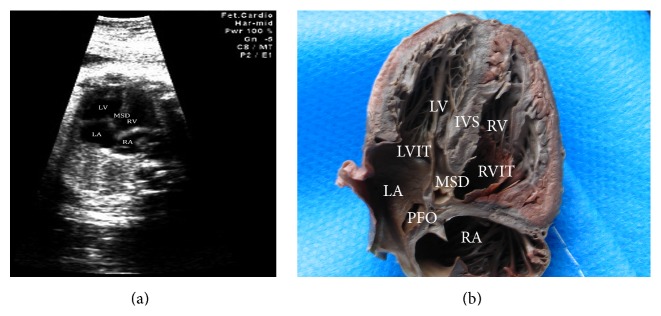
Ventricular septal defect (VSD) (32 w). (a) The apex four-chamber echocardiography section. (b) The apex four-chamber sectional anatomy. LA: left atrium; LV: left ventricle; RA: right atrium; RV: right ventricle; PFO: patent foramen ovale; IVS: interventricular septum; LVIT: left ventricular inflow tract; RVIT: right ventricular inflow tract; MSD: membranous septal defect.
